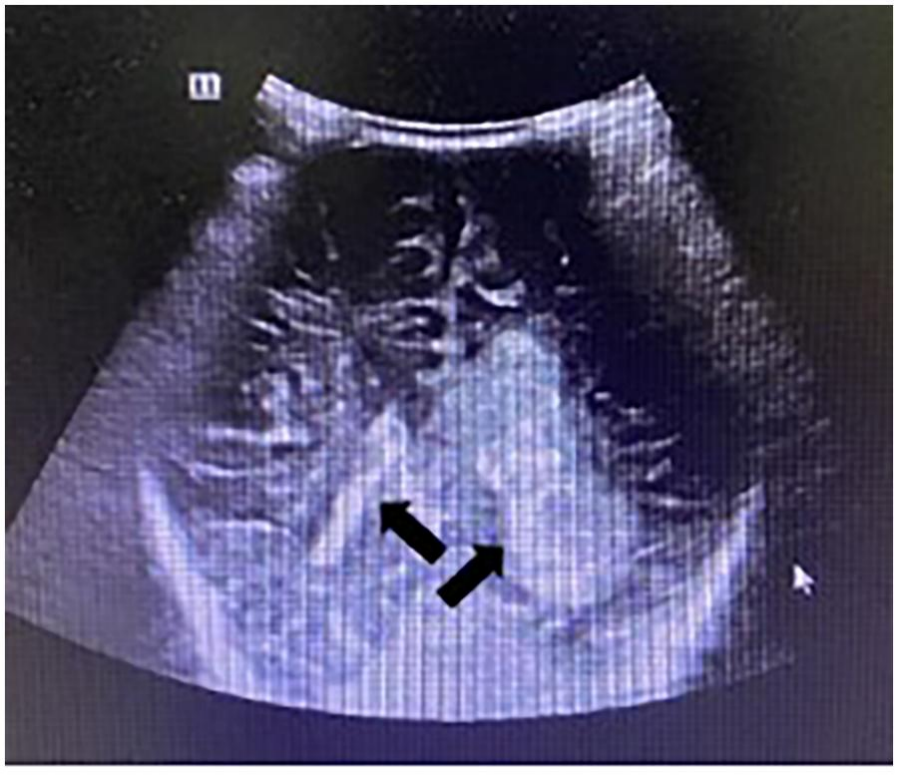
Cranial ultrasonography. Imaging findings revealed a patchy hyperechoic area in the left lateral ventricle and posterolateral region.
